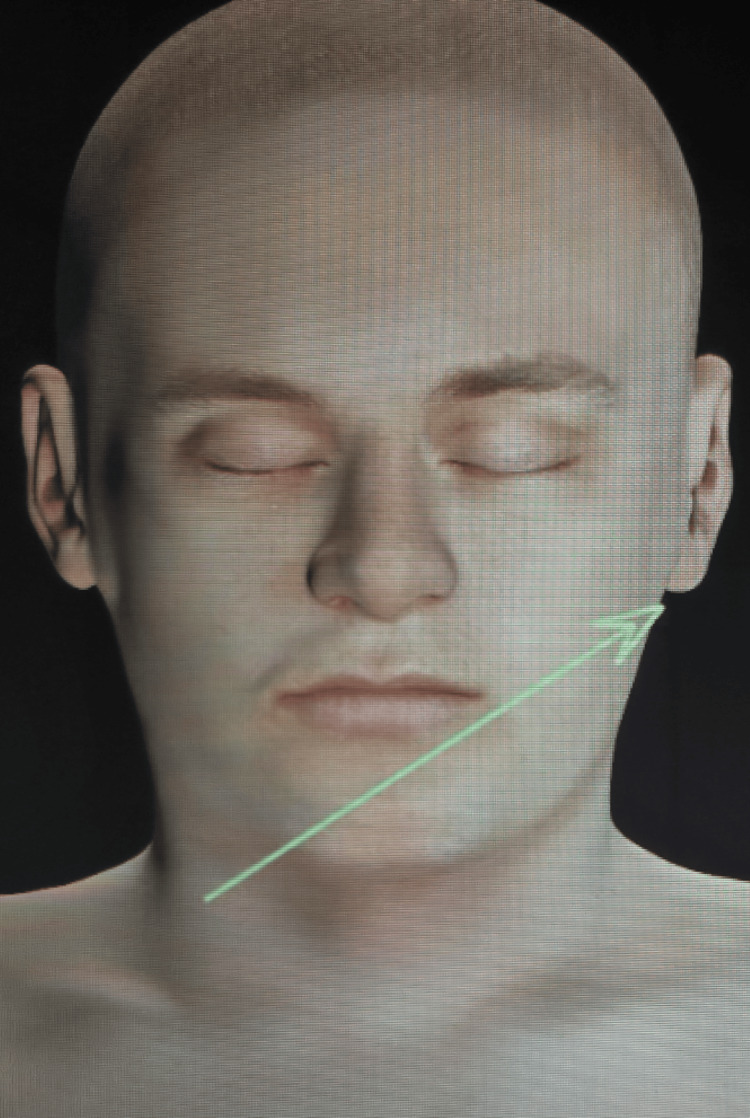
Depiction of the wound tract on the body surface 3D images were created from DICOM images of CECT scans of the head, neck, and thorax using the Anatomage table to determine the bullet's direction of the bullet. CECT: contrast-enhanced computed tomography
